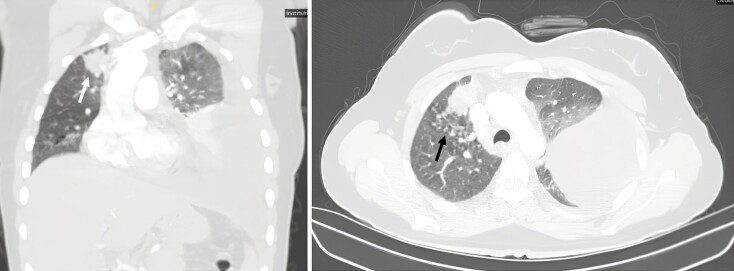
Figure 1. Computed tomography of thorax
